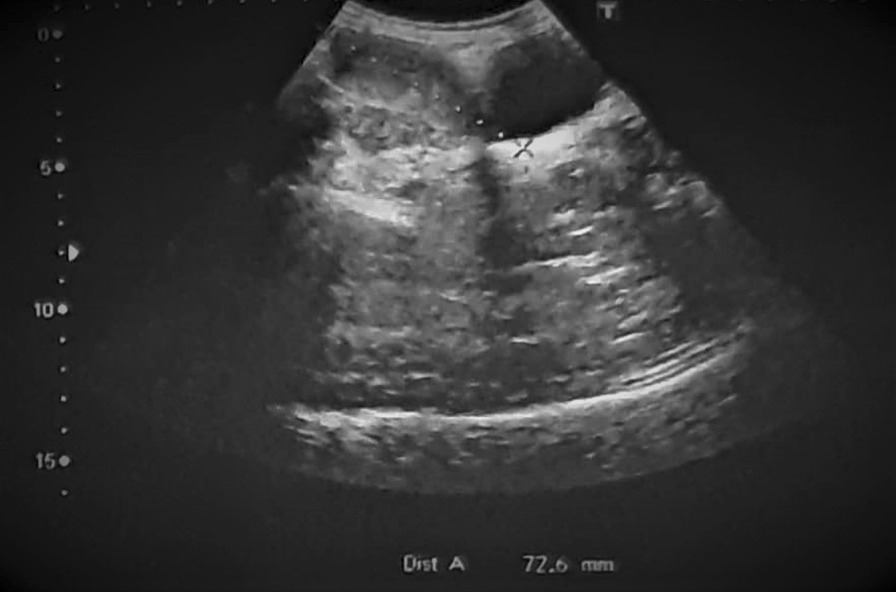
Pelvic ultrasonography demonstrating a large ovarian mass measuring 154 × 104 mm
